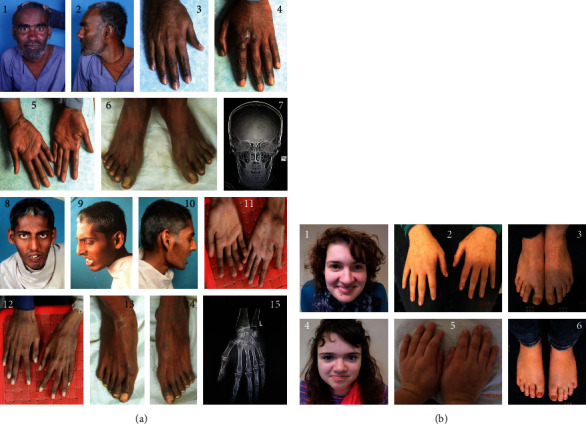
Photographs depicting the craniofacial and limb features of individuals with biallelic variants in *PGAP2*. (a) Photographs of family 1, featuring elder brother V-2 in photos 1 to 7 and his younger brother V-4 in photos 8 to 15. Subject V-2 displays macrocephaly and some facial asymmetry (1). He also presents fluent eyebrows with synophrys and a tendency towards telecanthus/hypertelorism (1) as well as an appearance of bifid tragus (2). Brachydactyly of the 5th fingers is observed (3, 4, and 5), along with tapering fingers (3 and 4). A bifurcation of the transverse proximal palmar flexion crease is seen in the palm (5). Camptodactyly in the 3rd left toe and 5th right toe is evident (6). X-ray of the head confirms macrocephaly (7). Subject V-4 presents macrocephaly with a triangular-shaped head, mild synophrys, telecanthus with hypertelorism, and distinctive eyes (small irises compared to enlarged eyeballs) (8, 9, and 10). Hypoplasia of the thenar areas is observed in the palms with no apparent vertical palmar flexion creases (11). Bilaterally, there is minor syndactyly between fingers 2 and 3 and tapering fingers (12), and camptodactyly of the 5th right toe is present (13). X-ray of the left hand exhibits mild soft tissue syndactyly between the 2nd and 3rd fingers, as well as between the 3rd and 4th fingers (15). Similar to his elder brother V-2 (X-ray not shown), the distal phalanges of the 2^nd^, 3^rd^, and 4^th^ fingers also appear slightly shorter (15). (b) Photographs depicting family 2 show the elder sister, IV-1, in photos 1 to 3, and her younger sister, IV-2, in photos 4 to 6. The elder sister IV-1 exhibits a prominent forehead with a pointed chin, along with telecanthus/hypertelorism (1). Dorsal view of the hands reveals bilateral shortening of the 5th fingers along with tapering fingers (2). Dorsal view of the feet shows a bilateral tendency to camptodactyly in toes 4 and 5 (3). The younger sister IV-2 exhibits similar facial features, including a prominent forehead, pointed chin, telecanthus/hypertelorism, and a broad nose with a wide nasal tip (4). Both hands show tapering fingers and noticeable shortening of the 5th finger (5). Dorsal view of the feet reveals a gap between the halluces and 2nd toes, along with a tendency towards clino/camptodactyly of toes 4 and 5, and possible syndactyly of toes 2 and 3 on the right foot (6).
